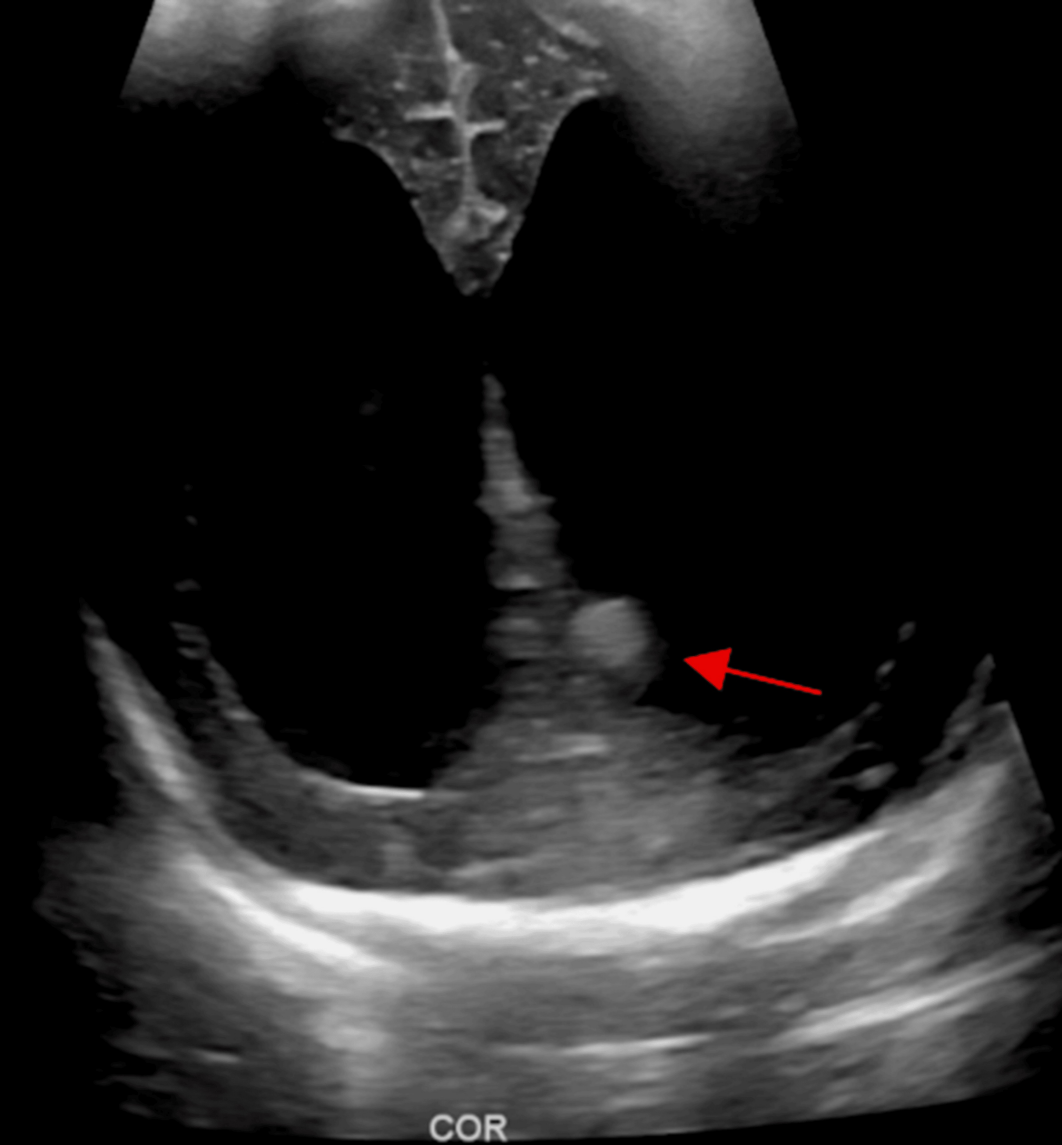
Ultrasonography of twin female with hyperechogenic mass in left lateral ventricle (red arrow) and ventriculomegaly of the lateral ventricles.
